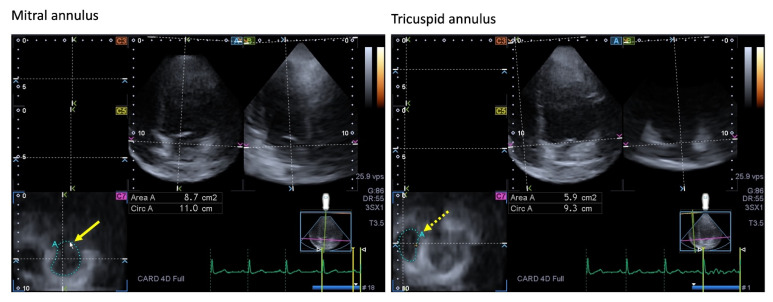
Images showing two-dimensionally projected views of the mitral (MA) and tricuspid (TA) annuli as assessed by three-dimensional (3D) speckle-tracking echocardiography. After the acquisition of the 3D echocardiographic dataset, the following views were produced: Apical four-chamber (A) and two-chamber views (B) and a cross-sectional view at the level of the MA/TA were optimized in apical four- and two-chamber views (C7). The yellow arrow represents the two-dimensional projection of the MA plane, whereas the yellow dashed arrow represents the two-dimensional projection of the TA plane. Abbreviations: LA = left atrium, LV = left ventricle, RA = right atrium, RV = right ventricle, Area = MA/TA area, Circ = MA/TA perimeter.
